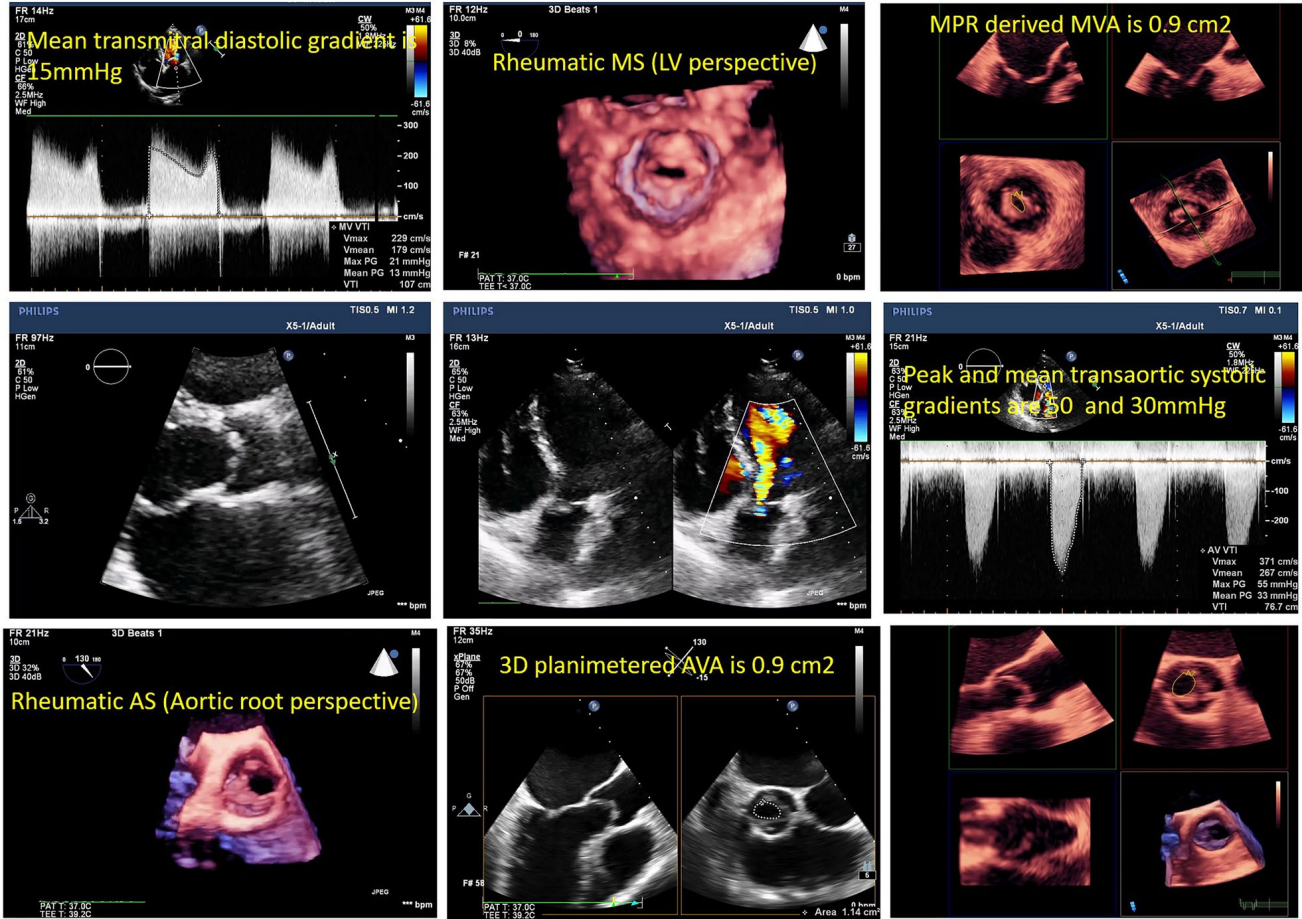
A case of rheumatic mitral and aortic valve diseases. In the upper panel, severe mitral valve stenosis was found; mean diastolic gradient = 15 mmHg .3D zoomed volume shows the characteristic "en-face" view of the thickened leaflets and commissural fusion. MVA was traced using MPR as 0.9 cm2. In the middle panel, a 2D parasternal long-axis view of the aortic valve shows systolic doming of the cusps. The apical 5-chamber view shows aortic valve regurgitation. In the lower panel, 3D-zoomed volume of the aortic valve shows thickened cusps and fused commissure between right and non-coronary cusps. X-plane mode and MPR-derived AVA are 0.9 cm2
